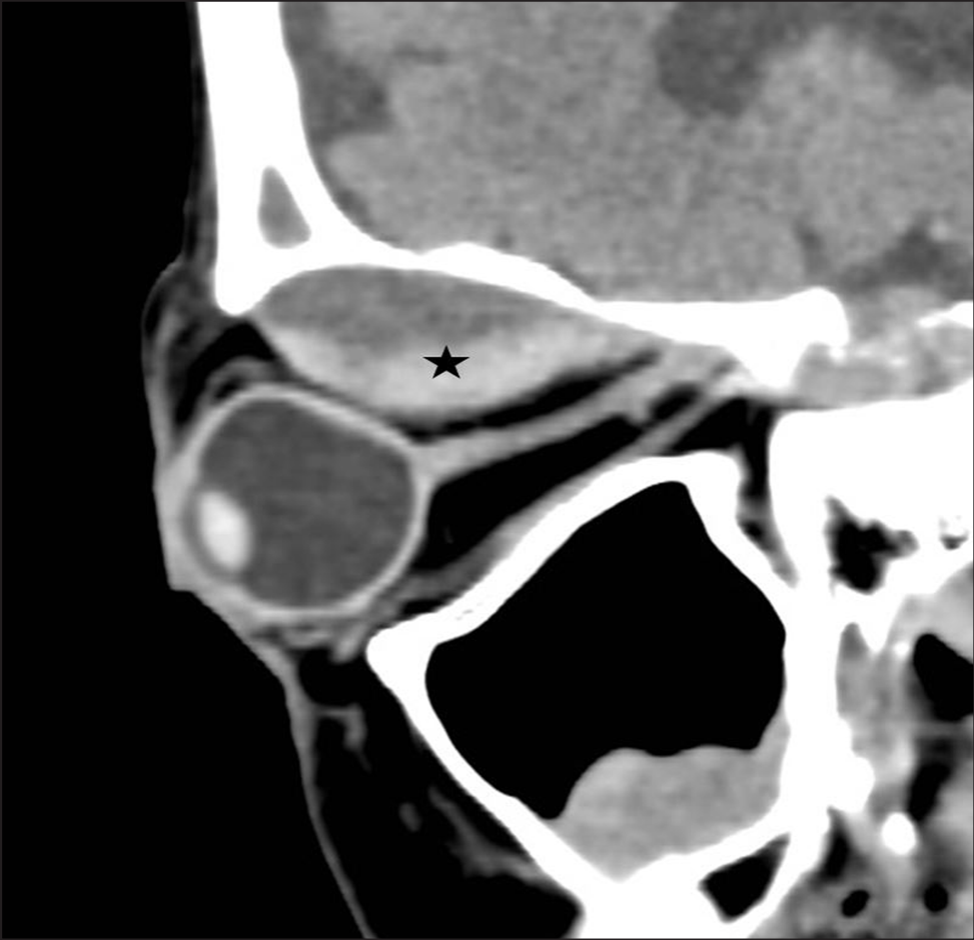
Magnetic resonance imaging (MRI) indicated an acute to subacute hematoma with hypointense signal on T2/FLAIR-weighted images (Figure 3a and b, arrows) and isointense to hyperintense signal on T1-weighted images (Figure 3c). Diffusion-weighted imaging was perturbated, as usual in a hematoma (Figure 3d). A subtle peripheral enhancement was noted after Gadolinium administration, probably caused by a reactional inflammation. MRI also confirmed the absence of infiltration of the retro-orbital fat and excluded a cerebral venous sinus thrombosis. Optic nerve was normal.
